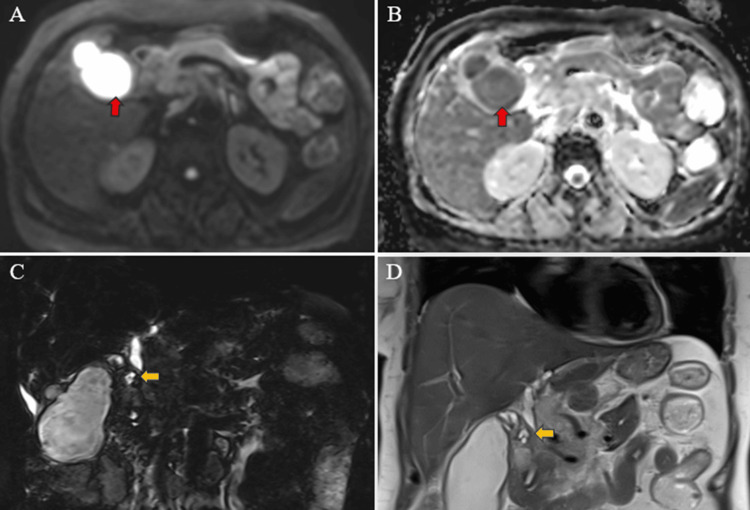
MR abdomen and MRCP images depict the gallbladder collection and pericholecystic collection (red arrow) showing restricted diffusion on DWI (A) and corresponding low ADC values observed in the same gallbladder and pericholecystic collection (red arrow) (B). Additionally, narrowing in the mid-CBD (yellow arrow) with dilated proximal CBD is evident on T2WI SPACE (C) and T2WI HASTE (D) images. MR: Magnetic resonance; MRCP: MR cholangiopancreatography; T2WI: T2-weighted imaging; DWI: diffusion-weighted imaging; ADC: apparent diffusion coefficient; SPACE: sampling perfection with application optimized contrast using different flip angle evolution; HASTE: half-Fourier single-shot turbo spin-echo
